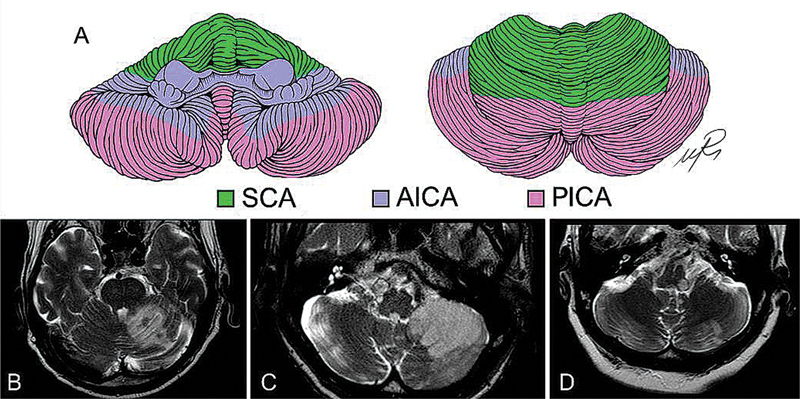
Abbreviations: AICA, anterior inferior cerebellar artery; FLAIR, fluid-attenuated inversion recovery; SCA, superior cerebellar artery; PICA, posterior inferior cerebellar artery.
